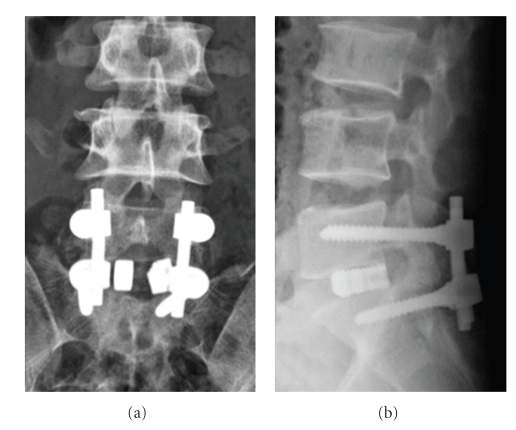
Postoperative anteroposterior (a) and lateral (b) radiographs showing good reduction of L5 anterolisthesis.
